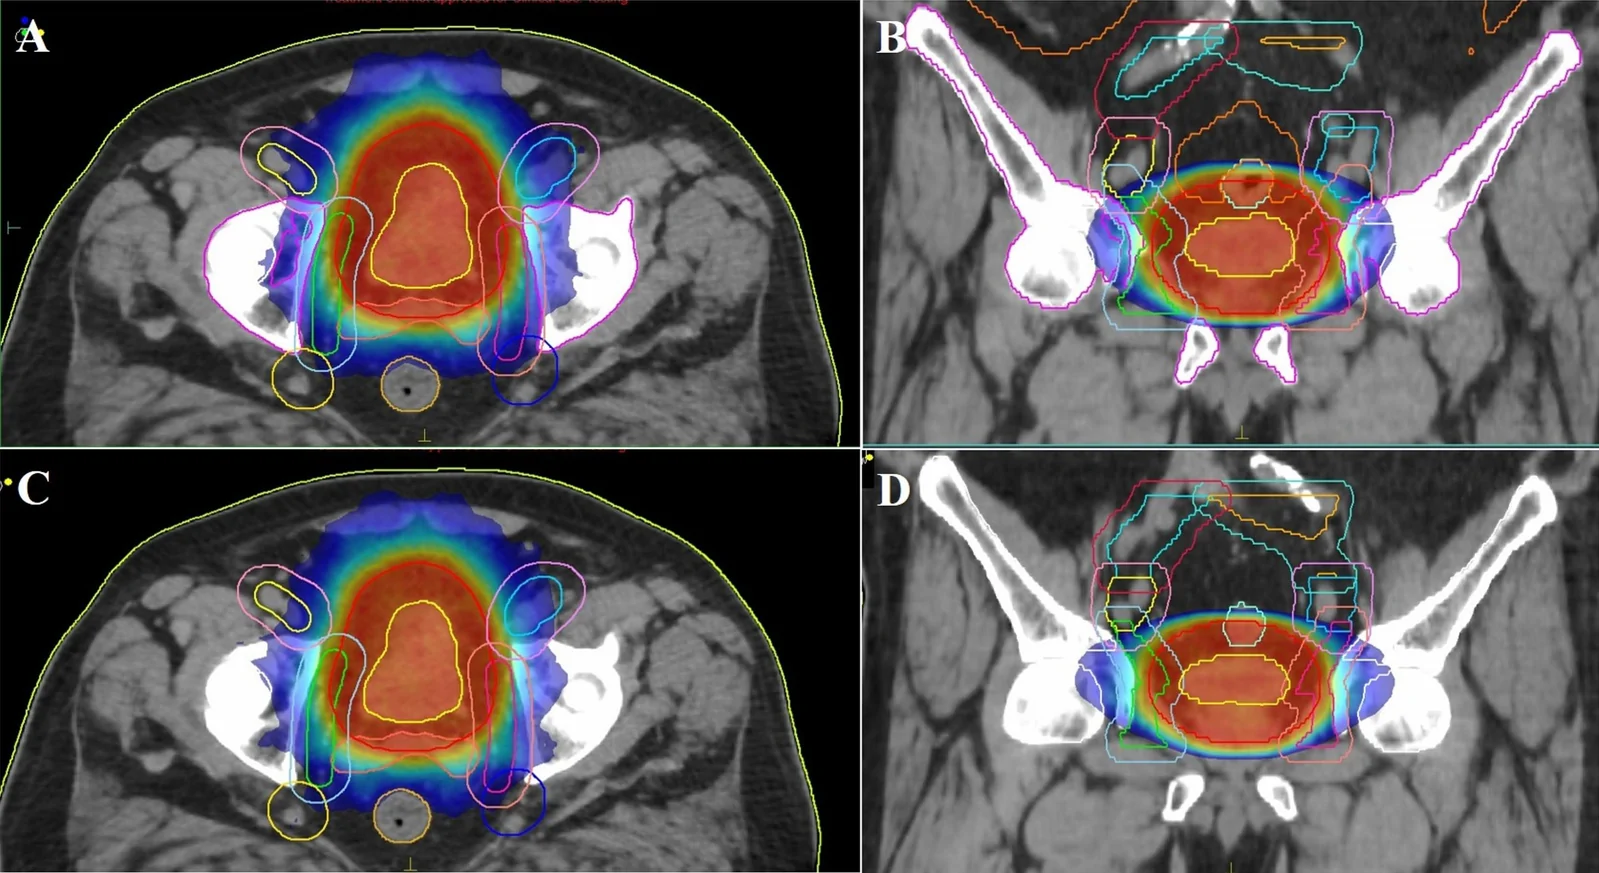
Representative axial and coronal computed tomography images showing 50% dose distributions of prescribed dose and contoured bladder target and pelvic nodal subregions for VMAT (A, B) and step-and-shoot IMRT (C, D).
